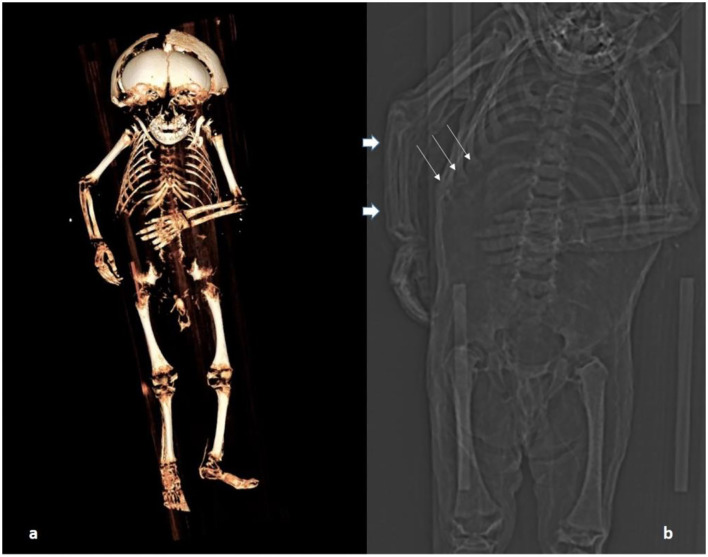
CT scan of the body: (a) Three-dimensional reconstruction of the skeleton. (b) A section of the topogram showing particularly the rosary of the costochondral junction (thin arrows); long bones are straight (possibly the right ulna is very slightly bent; thick arrows) and the metaphyses are minimally enlarged at upper and lower long bones.
